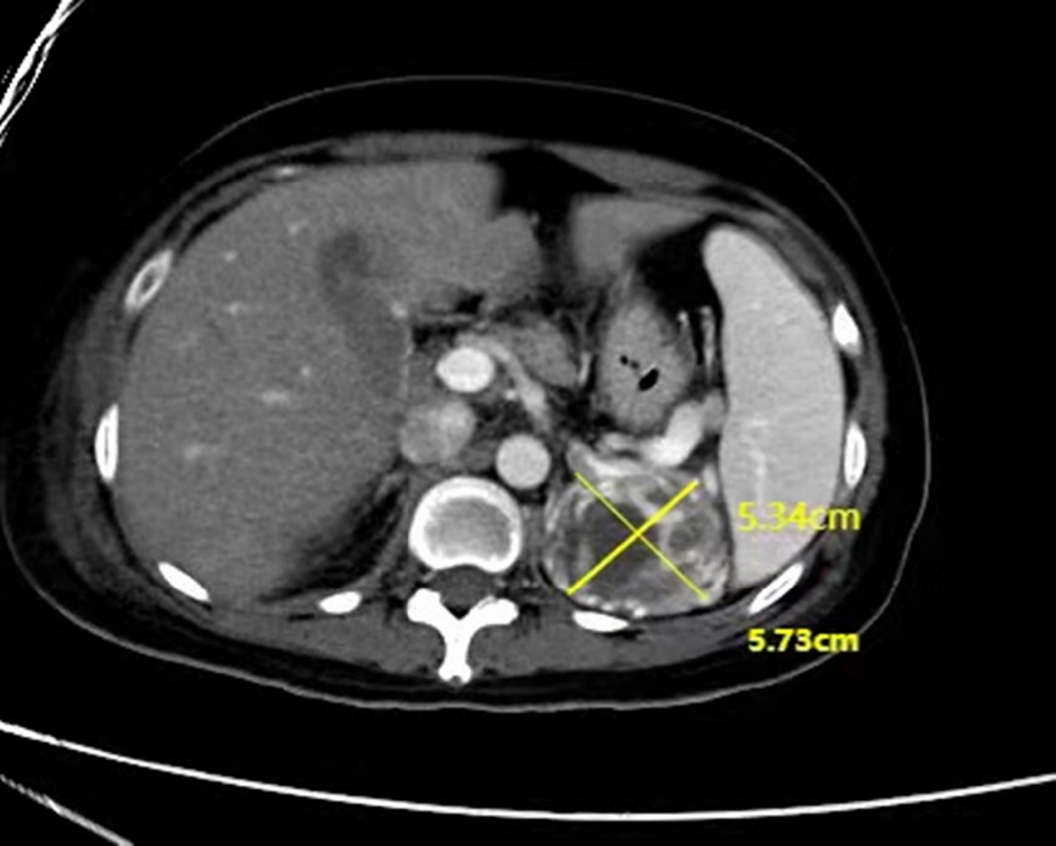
Results of renal CT examination. The arrow points to the left adrenal mass, the size is 5.34 × 5.73 cm.
